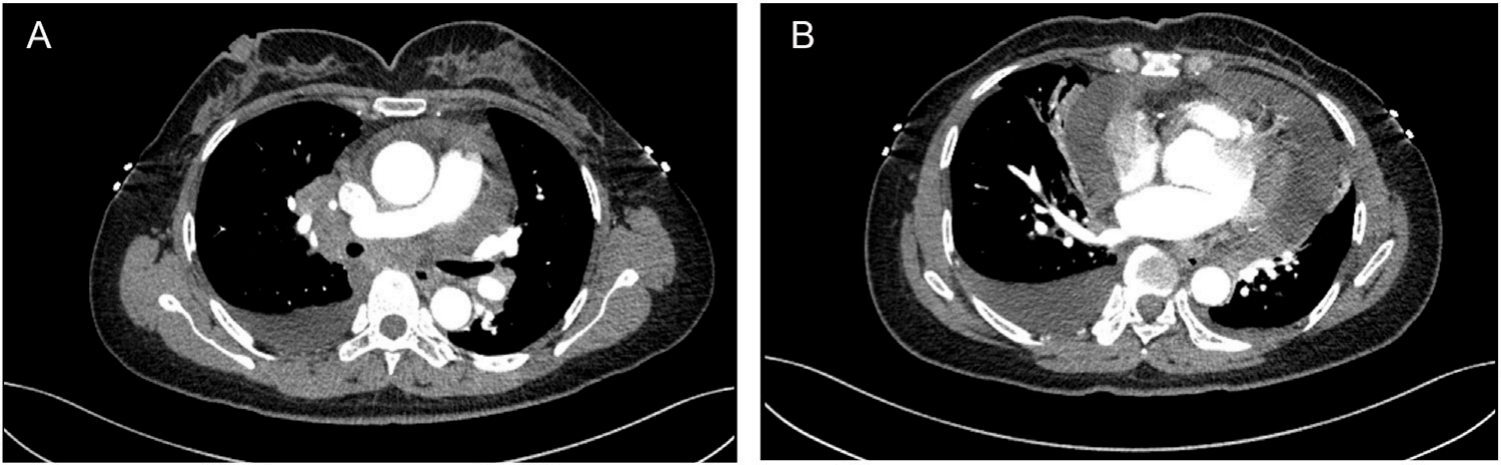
Chest computed tomography revealed a right hilar mass (A), pleural and pericardial effusion (B).
